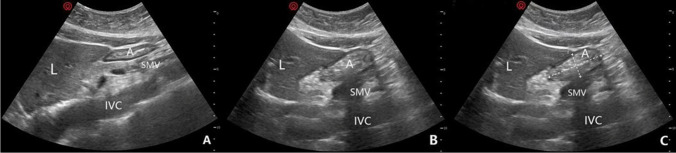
Ultrasonographic images of the gastric antrum. Images in the supine position (A). Images in the right lateral decubitus position (RLDP) (B). Anterior-posterior (AP) and craniocaudal (CC) diameters of the gastric antrum were measured in the RLDP to calculate antral cross-sectional area (C). A = antrum, IVC = inferior vena cava, L = liver, SMV = superior mesenteric vein
